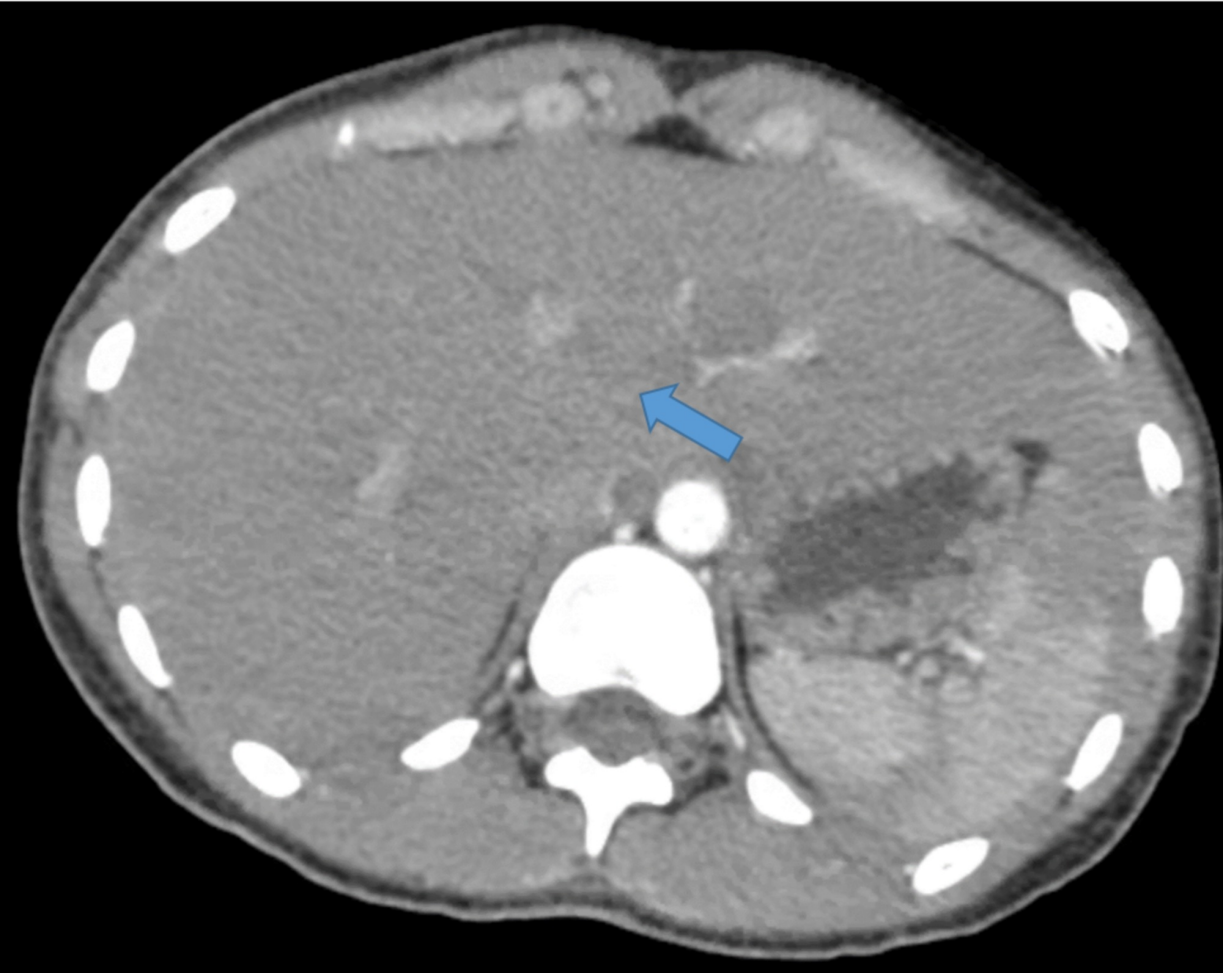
Computed tomography angiography (CTA) chest: Hepatomegaly, with generous left lobe wrapping laterally around the spleen. Mild upper abdominal ascites particularly in the perihepatic region.
